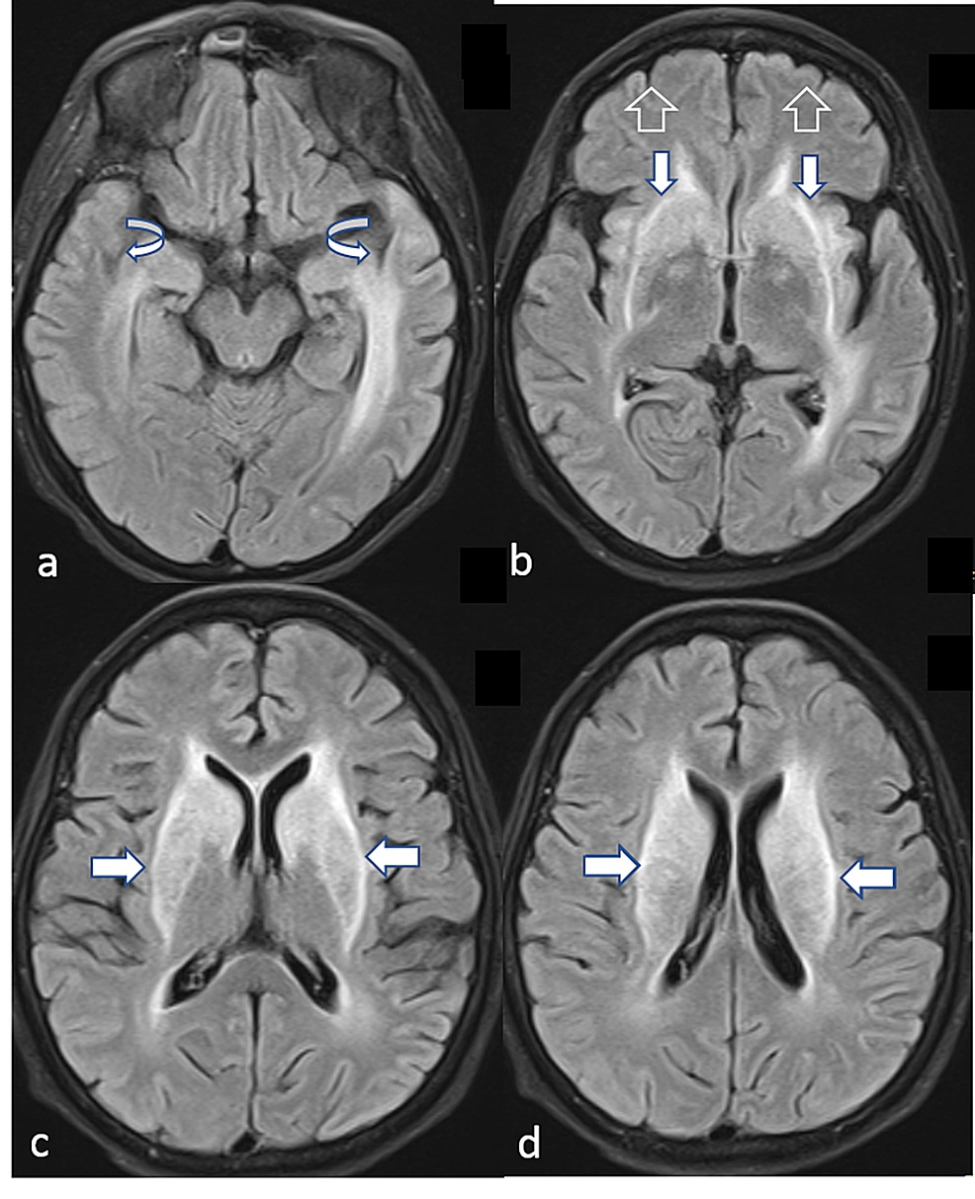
Follow-up MRI axial FLAIR images. (a-d) show mild regression of the hyperintense areas mainly at previously seen subcortical and external capsule regions (denoted by the horizontal, vertical, hollow, and curved arrows). FLAIR, fluid-attenuated inversion recovery
